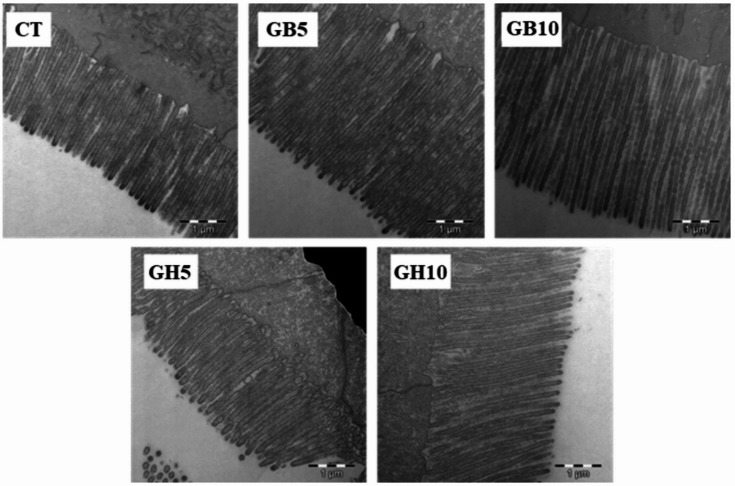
TEM details of the apical surface of the enterocytes in gilthead seabream juveniles fed the different experimental diets (TEM. Scale: 1 μm). Dietary codes: CT: control; GB5: 0.5% inclusion of LB-GREENboost; GB10: 1% inclusion of LB-GREENboost; GH5: 0.5% inclusion of LB-GUThealth; GH10: 1% inclusion of LB-GUThealth.
